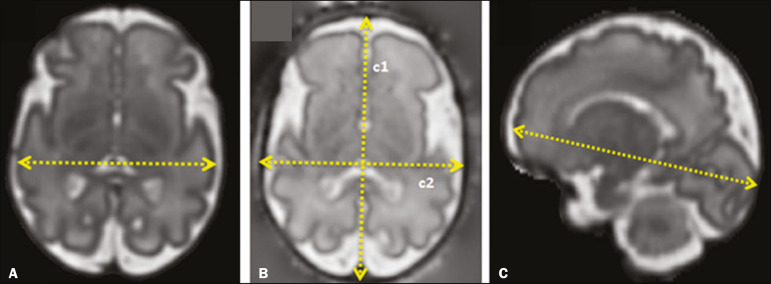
MRI measurements of brain BPD (A), skull BPD (B) and brain OFD (C).
